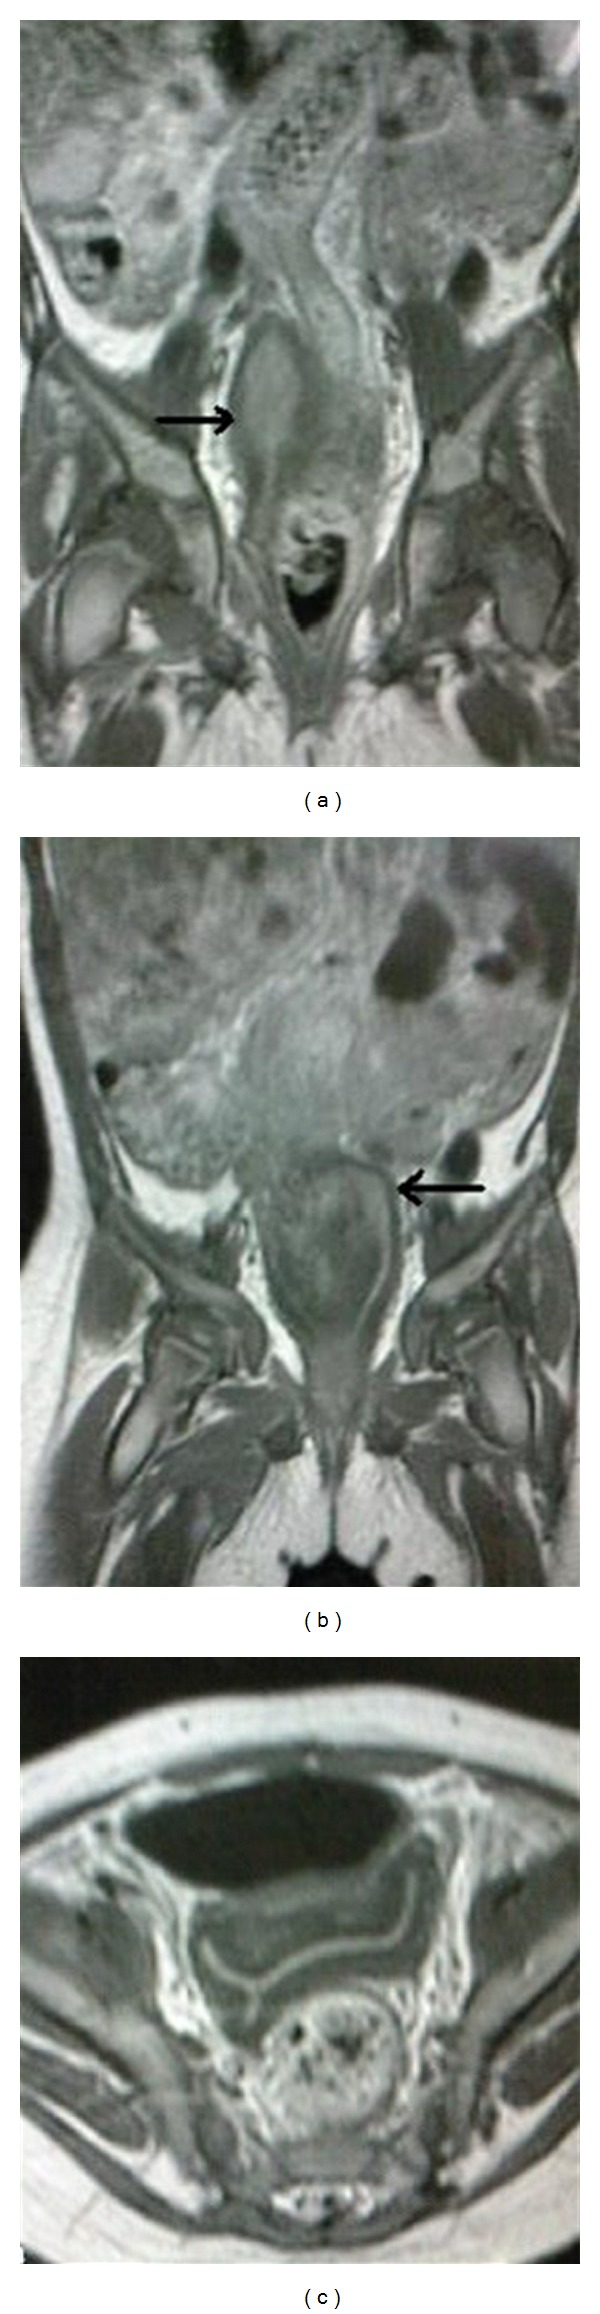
T1-weighed postoperative MR images. (a) and (b): two consecutive pelvic coronal images clearly show the two cavities (arrows) of the uterus separated by an incomplete longitudinal septum which was difficult to depict earlier. (c) axial view through corpus shows bicornuate uterus.
